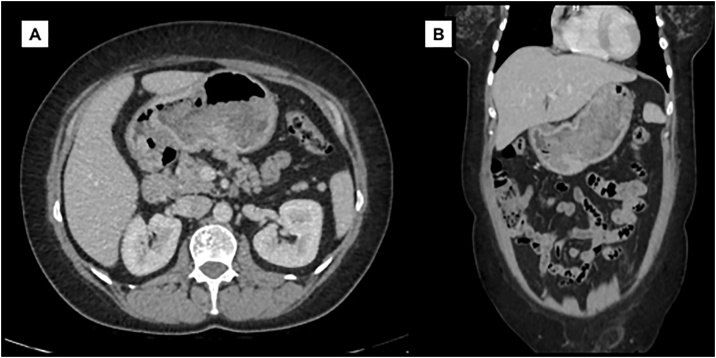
Abdominal computed tomography presenting diffuse gastric mucosa enhancement with no signs of extramural extension or abdominal lymphomegalies. The stomach appears bulky and filled with protruding luminal content from the mucosae (A, axial view; B, coronal view).
